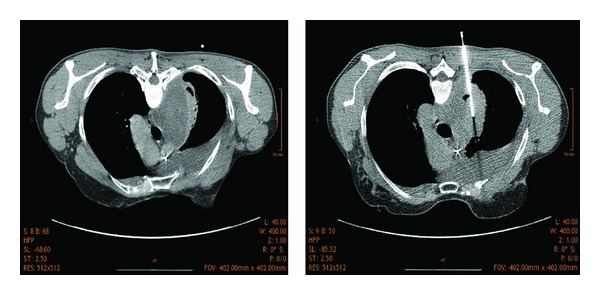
Computed tomography (CT) scan of the chest with CT-guided needle biopsy of a right paramediastinal tumor. In total 10 biopsies were taken from the 8.4 cm large mass.
